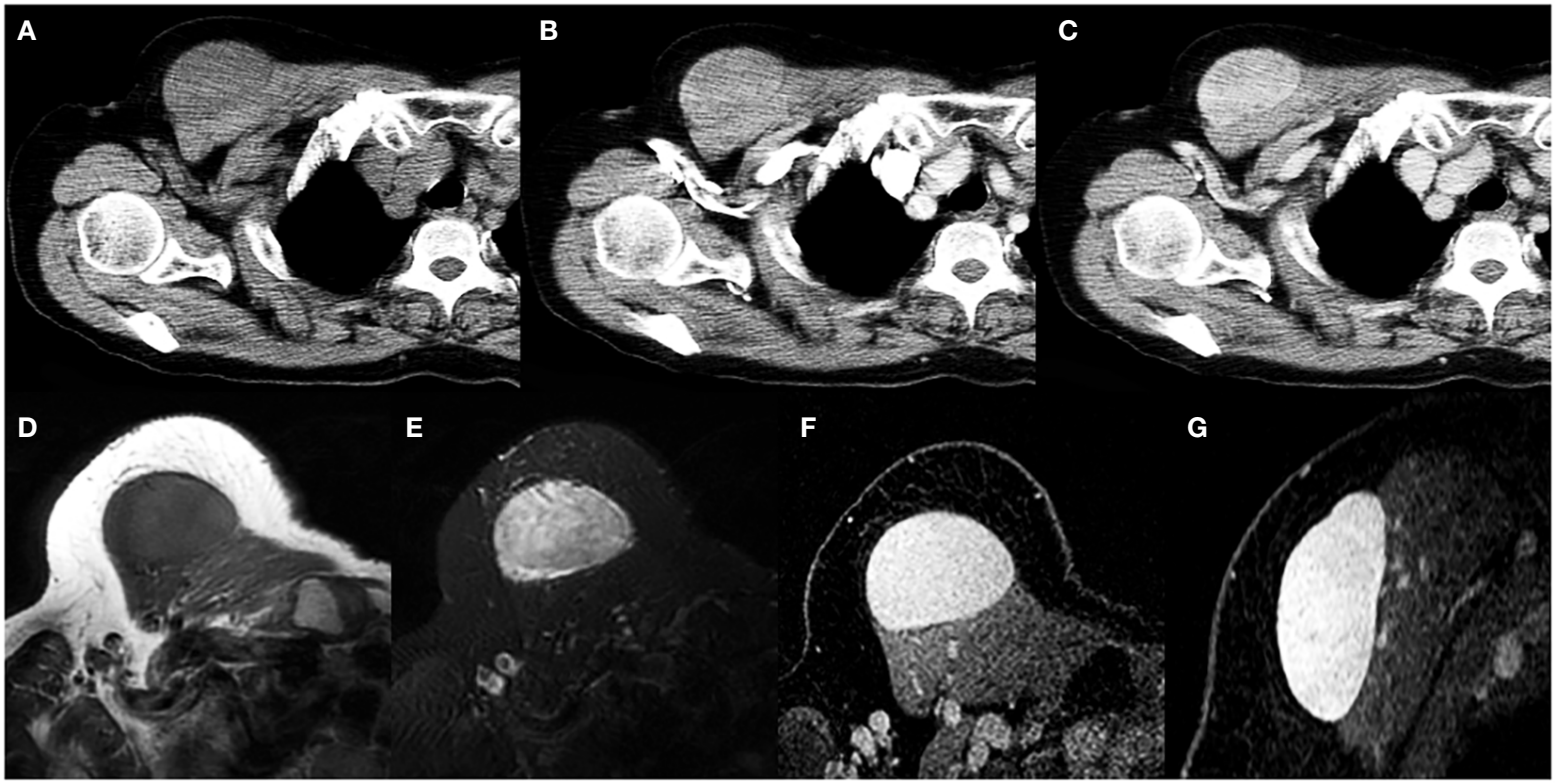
A 69-year-old woman with SFT. (A) Precontrast CT scan shows an oval mass in the anterior chest wall. (B) No obvious intratumoral vessels are seen in the arterial phase on post-contrast CT image. (C) Heterogeneous marked enhancement is detected in the venous phase. (D) The tumor shows low intensity on T1-weighted image. (E) A black-and-white mixed pattern is seen on T2-weighted image. (F, G) Heterogeneous moderate to marked enhancement are seen on postcontrast MR images.
